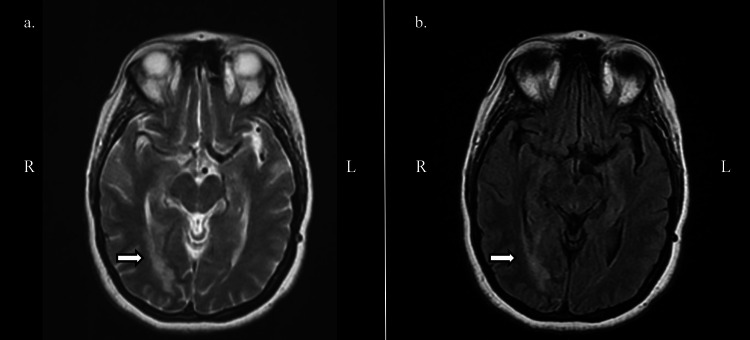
MR brain with and without contrast: superior view. Transverse superior view of MR brain (a) FSE and (b) FLAIR showing increased T2/FLAIR signal in the periventricular and subcortical white matter. MR: magnetic resonance, FLAIR: fluid-attenuated inversion recovery, FSE: fast spin echo.
